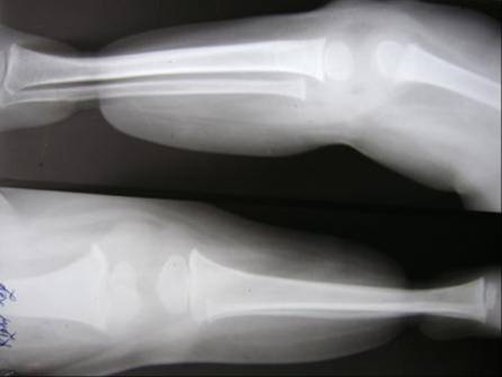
X-ray of both lower extremities showing severe soft tissue constriction with normal bone.
